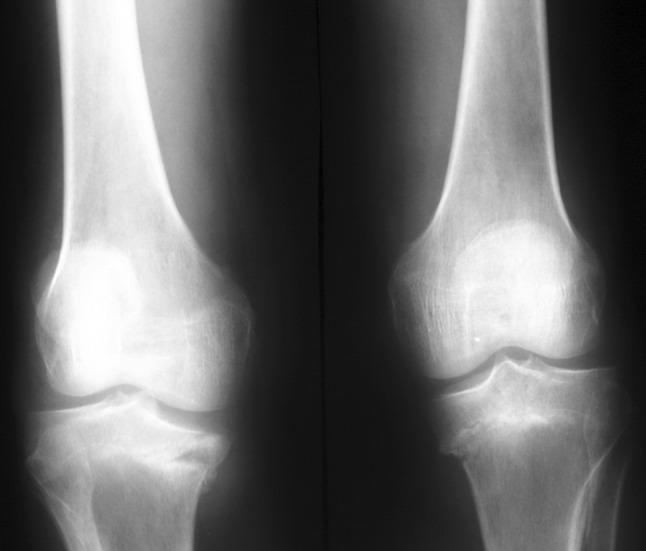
Radiographs of both knees at presentation
